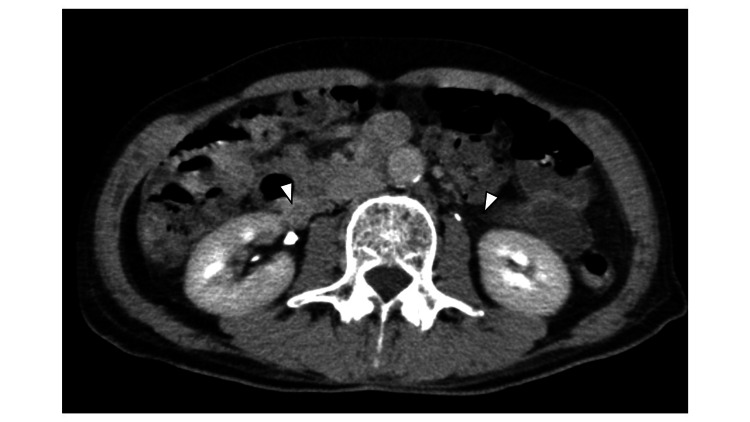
Follow-up axial contrast-enhanced abdominal CT imaging showing no ureteral overflow A follow-up contrast-enhanced CT was performed on day 9 and revealed no ureteral overflow (white arrowhead) CT: computed tomography
